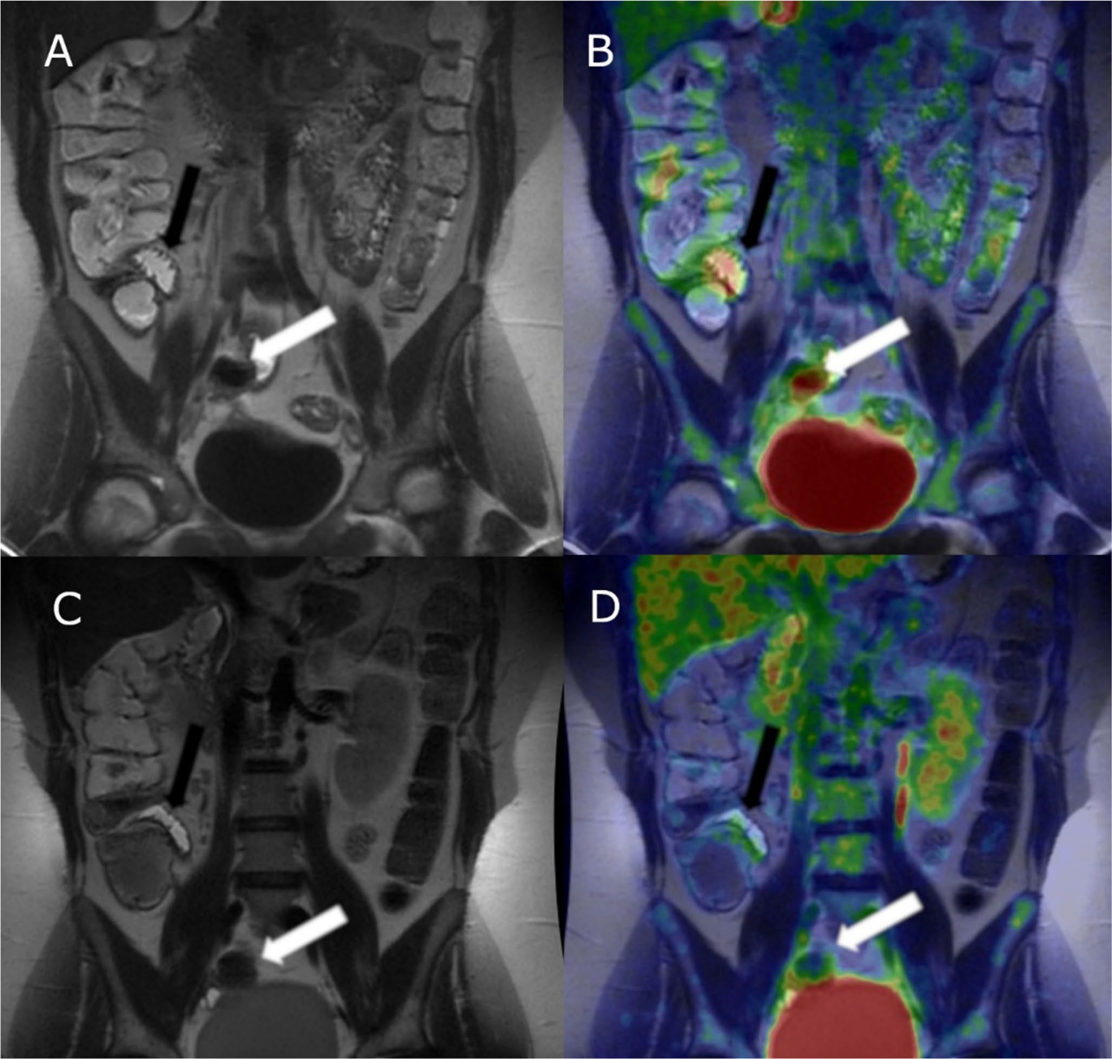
A 27-year-old female with small bowel CD related inflammation in terminal ileum (black arrows pointing at a distal skip lesion and white arrows pointing at a proximal skip lesion). In first diagnostic T2-weighted MRE inflammation is barely seen, sMARIA score (simplified Magnetic Resonance Index of Activity) 1 (A). In fused [^18^F]-FDG PET-MRE (B) inflammation is clearly seen in both segments, SUV_Max_ 6.6. Follow-up [^18^F]-FDG-PET-MRE was done 98 days after the diagnostic. No visible inflammation in T2-weighted MRE, sMARIA score 0 (C) and a clear decrease in inflammation is seen as [^18^F]-FDG-activity has subsided in fused [^18^F]-FDG PET-MRE, SUV_Max_ 1.3 (D)
